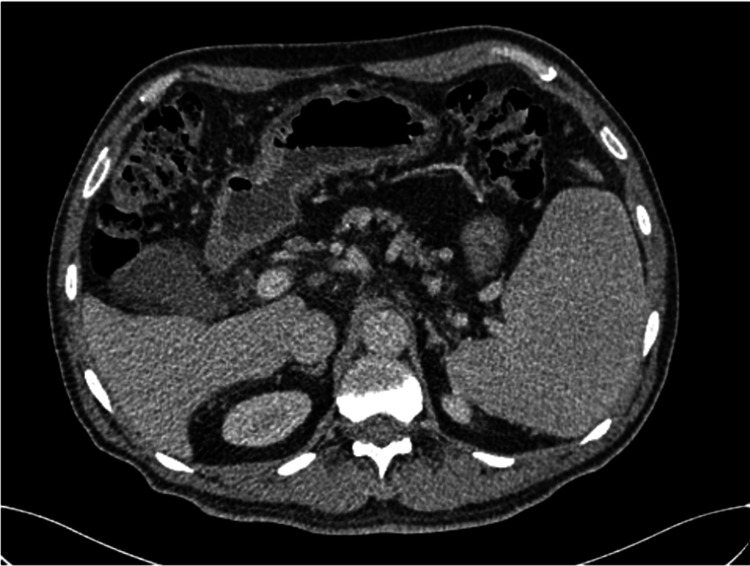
CT scan image shows no abdnormality in the stomach.
